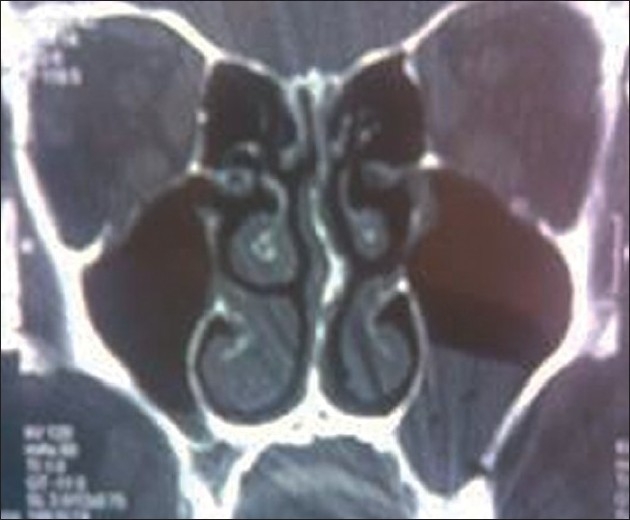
Left maxillary sinus accumulation in coronal CT
